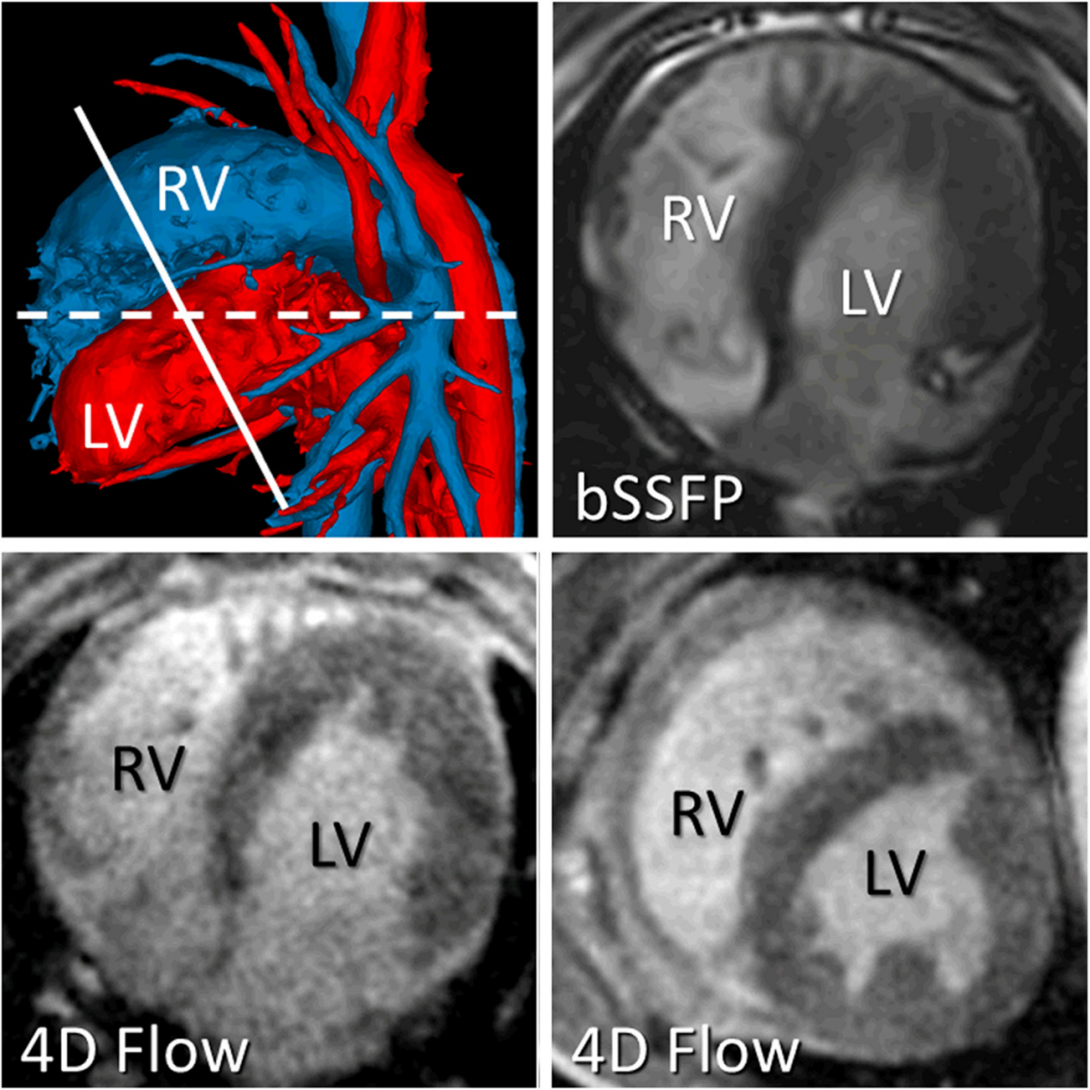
Surface-shaded angiographic image (top left) from 4D flow MRI dataset revealing right- (blue) and left- (red) sided circulations. Right (RV) and left (LV) ventricular volumes were calculated from bSSFP (top right) and 4D flow MRI magnitude (bottom left) images in the axial orientation (dashed line). In addition, the time-resolved 4D flow MRI magnitude dataset was reformatted into the LV short-axis orientation (bottom right and indicated by solid line in top left).
